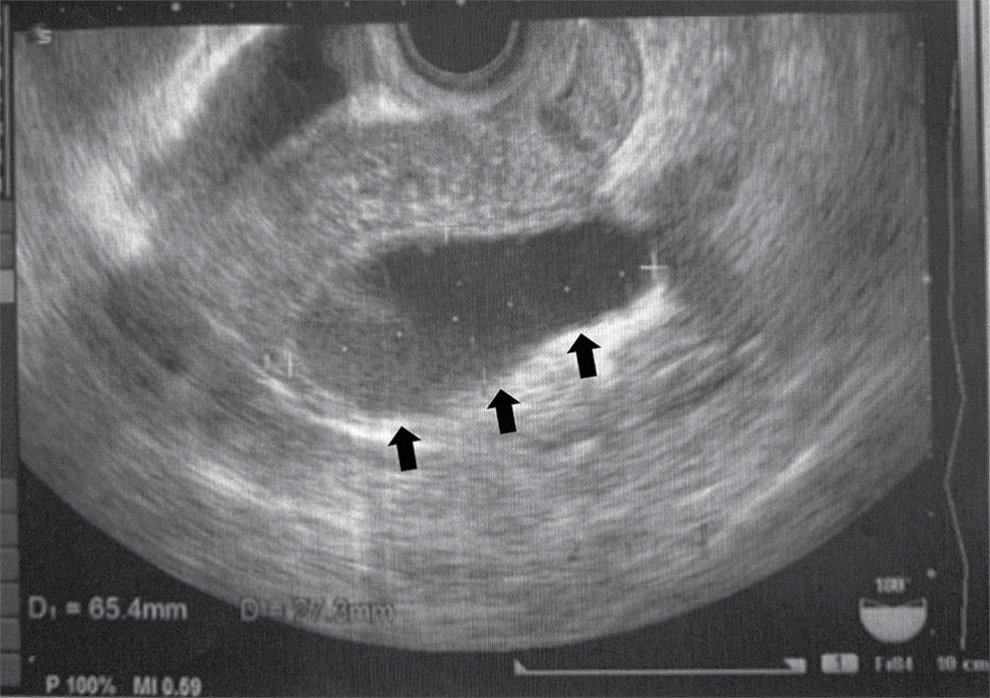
Transvaginal ultrasound on postoperative day 34 showing a hypoechoic cystic area adjacent to the uterus right posteriorly, measuring 65.4 mm × 27.3 mm.
